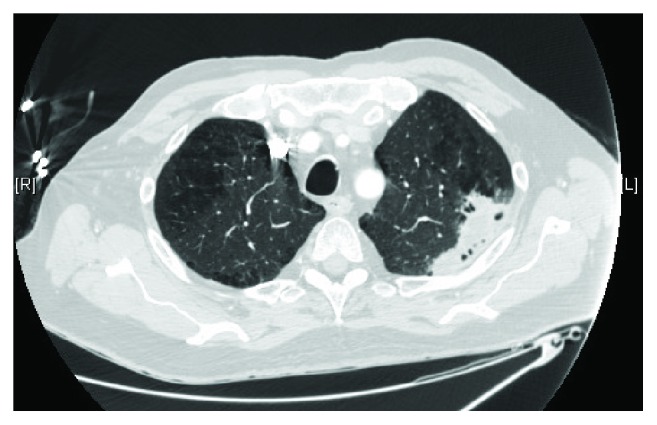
CT chest on presentation.
